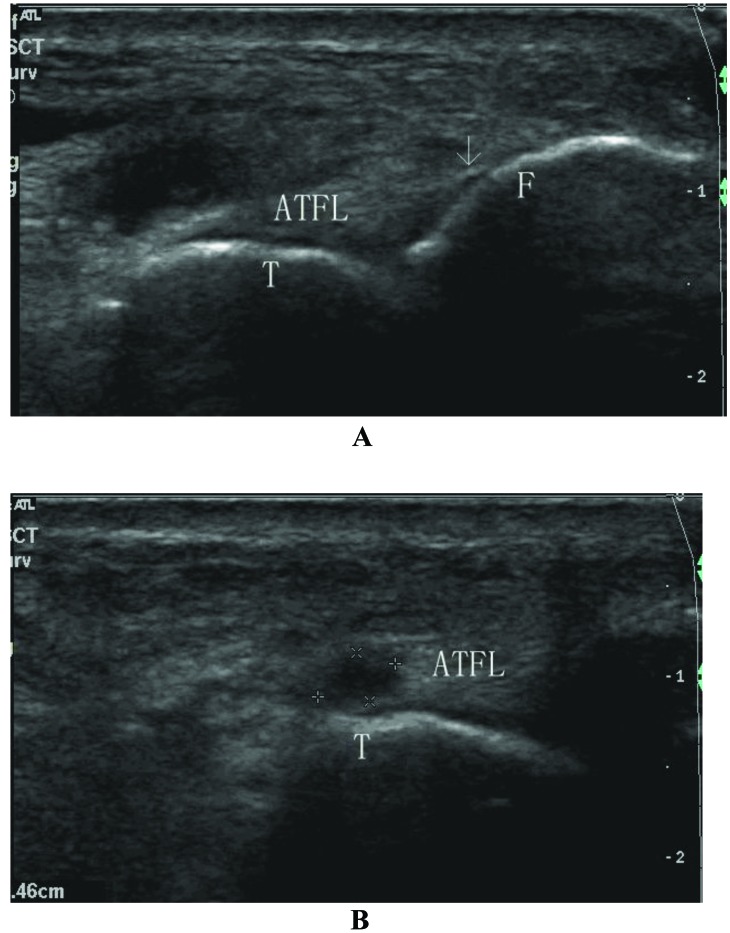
Sonogram shows the ligament injury at A) the fibular side (calcification) and B) the talar side (tear) (type VI). ATFL: anterior talofibular ligament; F: fibula; T: talus; Arrow: calcification at the fibular side; Star: ligament tear at the talar side.
